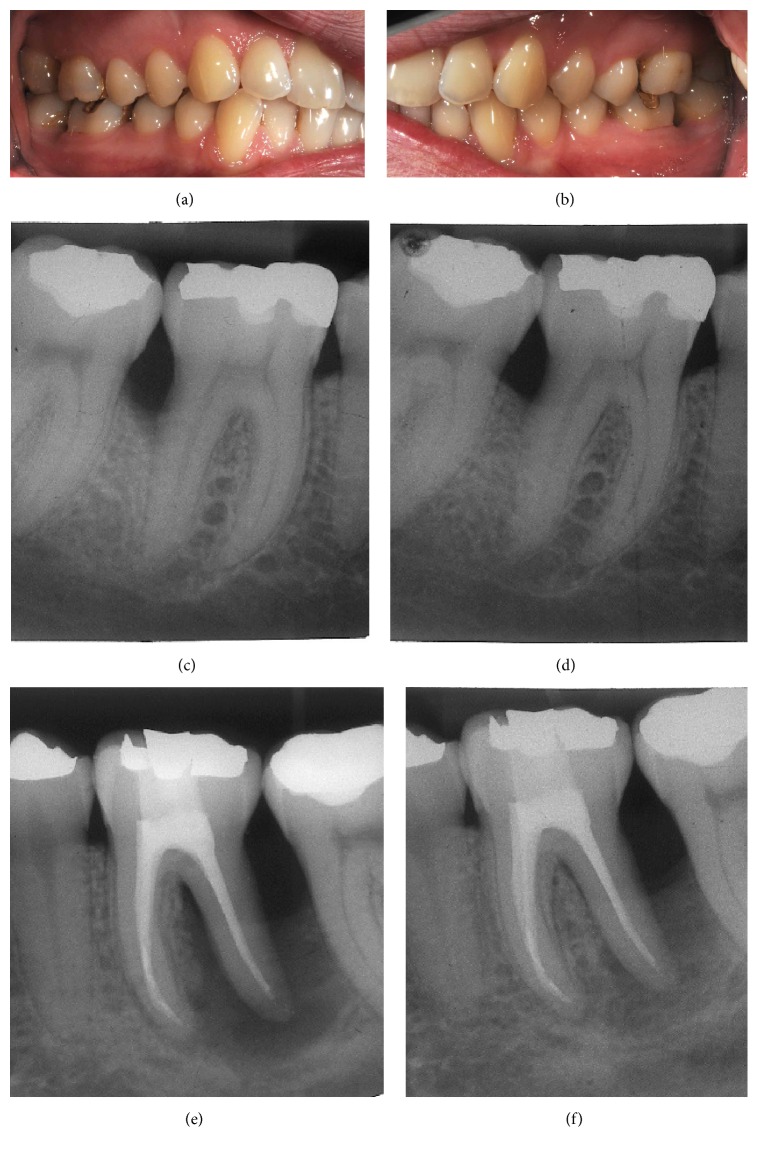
Clinical photographs (a, b) and radiographic images of teeth 46 (c, d) and 36 (e, f) at the 6- month reevaluation. Periodontal defects on both teeth demonstrated radiographic gain of bone structure in comparison to the baseline visit (c, e) 6 months after regenerative periodontal treatment (d, f).
